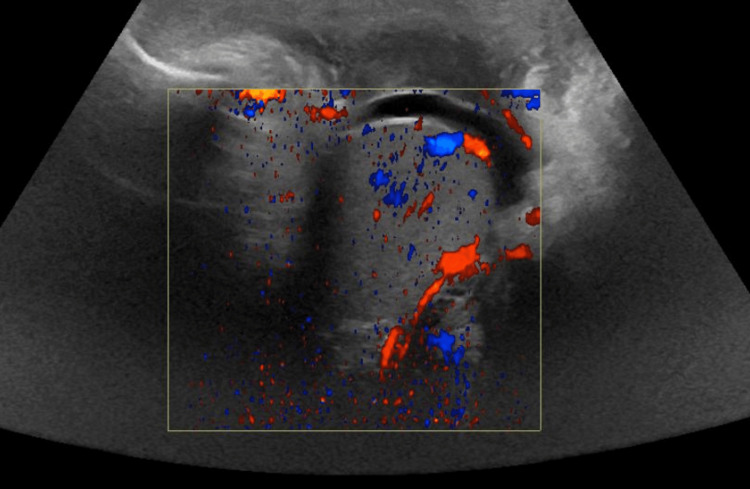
Reduced blood flow to the right testicle on Doppler ultrasound.
